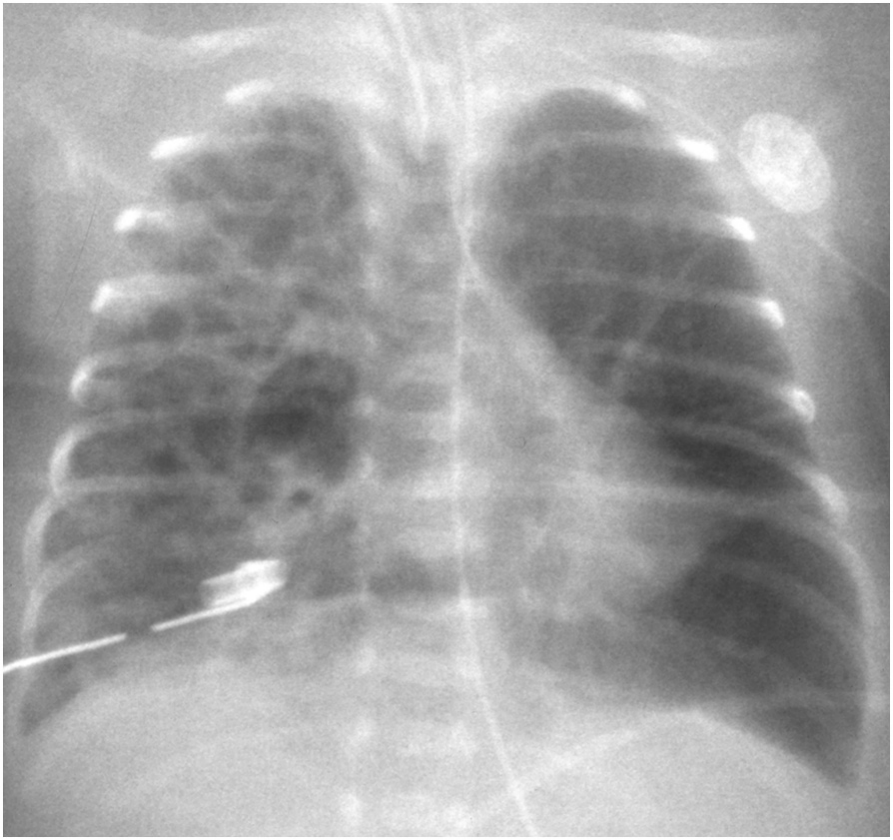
A chest X-ray obtained after lung puncture. Decompression of diffuse pulmonary interstitial emphysema was performed with a trocar catheter. The mediastinal shift improved after decompression.
